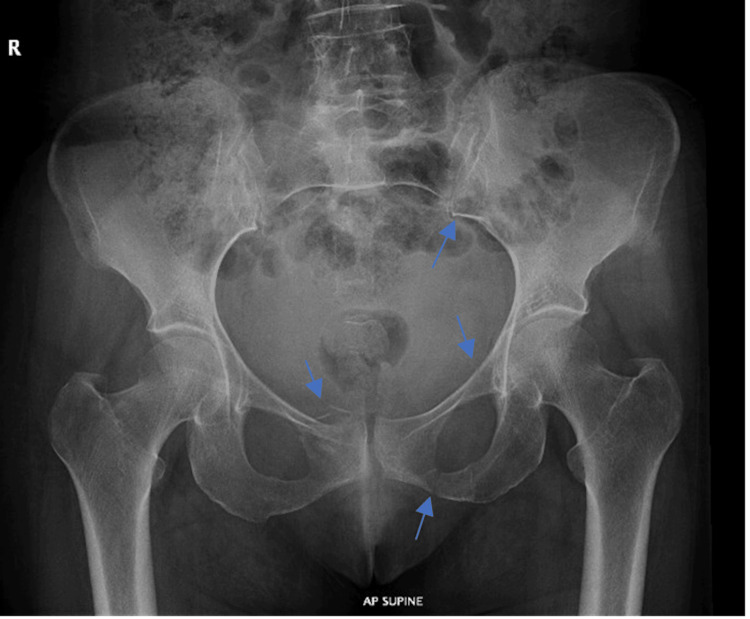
X-ray of the pelvis, showing a fracture of the left sacral ala, right SPR, left SPR, and inferior pubic rami (blue arrows) SPR: superior pubic ramus; AP: anteroposterior
